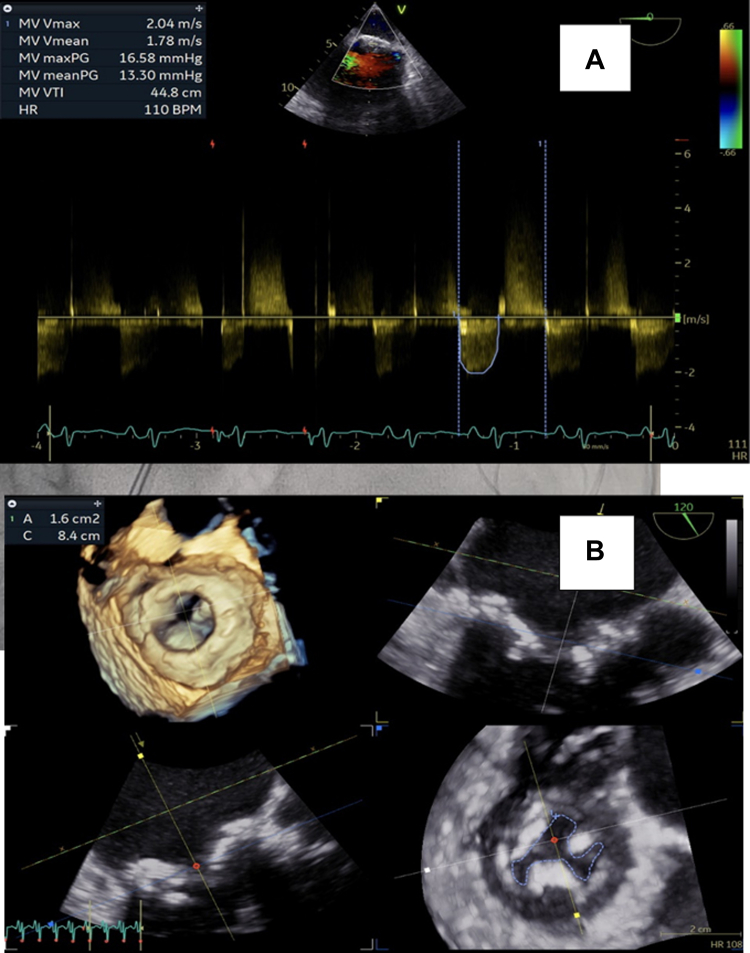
Echocardiographic Evaluation After Valvuloplasty (A) TEE 2-chamber view at 0°angle showing a mean transvalvular gradient of 13 mm Hg. (B) TEE 3D reconstruction demonstrating bioprosthesis in the mitral position after balloon valvuloplasty and orifice area measuring 1.6 cm^2^ using 3D planimetry. 3D = three-dimensional; TEE = transesophageal echocardiography.
